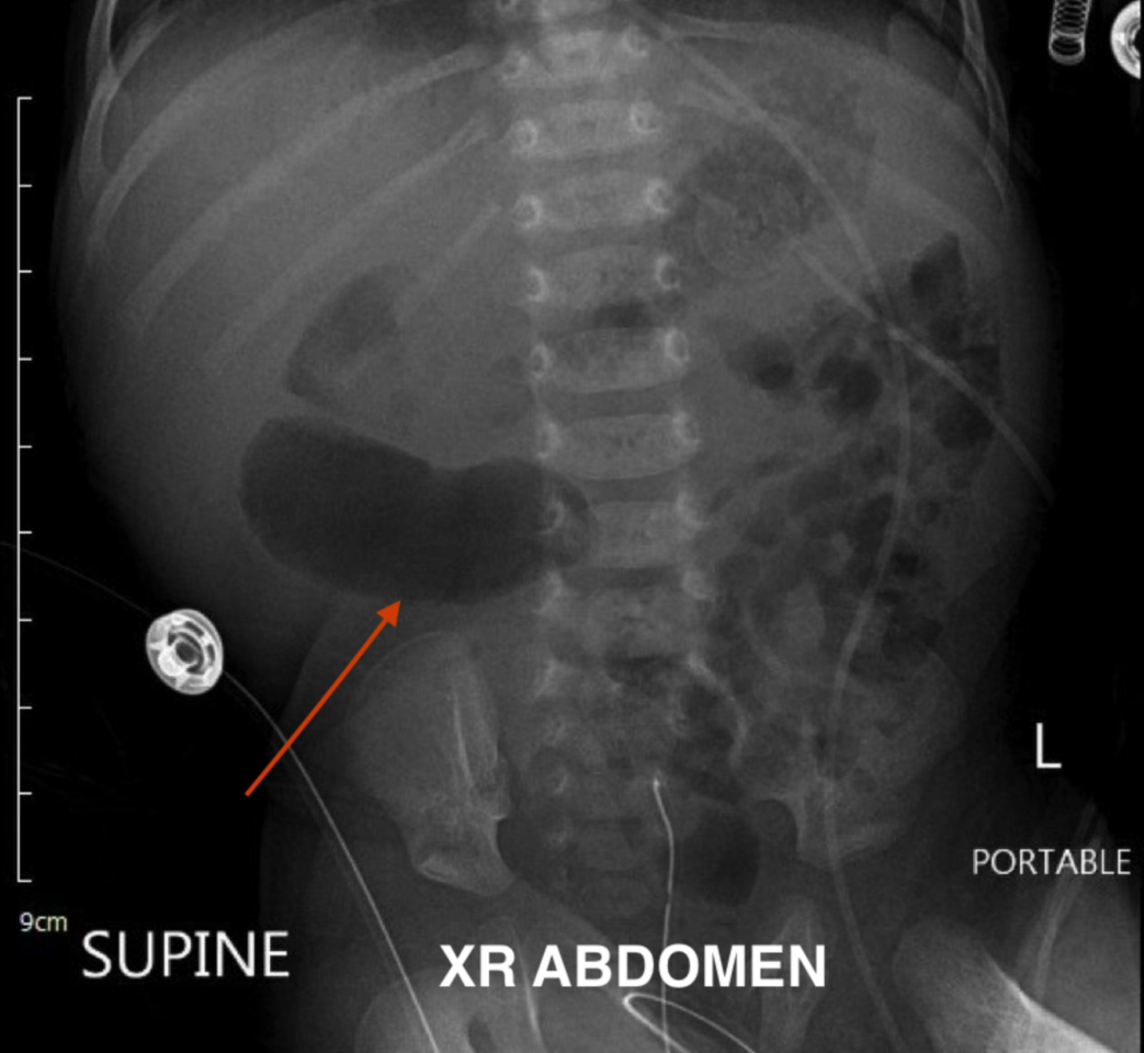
Abdominal X-ray with dialated loops of bowel
